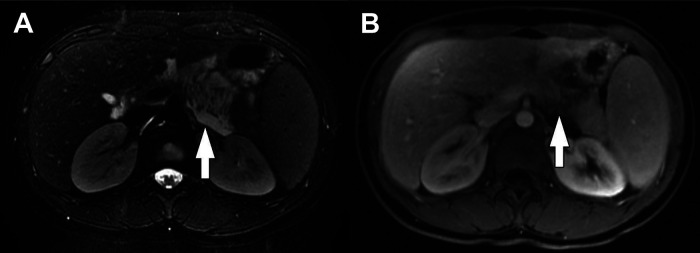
Lymphatic malformation. (A) Axial T2-weighted fat-saturated MRI and (B) Axial T1-weighted fat-saturated post-contrast MRI show an infiltrative lesion involving the body of the pancreas (arrows). The lesion is T2-weighted hyperintense reflecting fluid content without enhancement following contrast administration. Transspatial involvement is characteristic of a lymphatic malformation.
